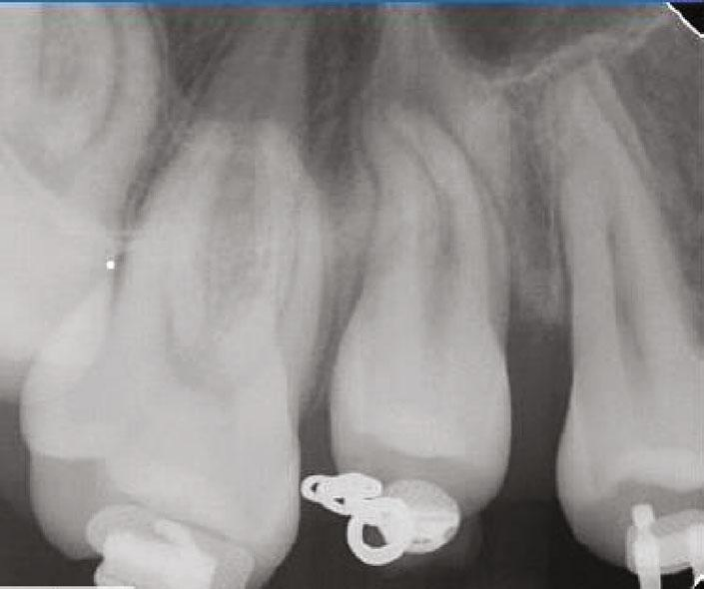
Enlarged follow-up periapical radiograph tooth #4, 6 months postsurgical exposure.
